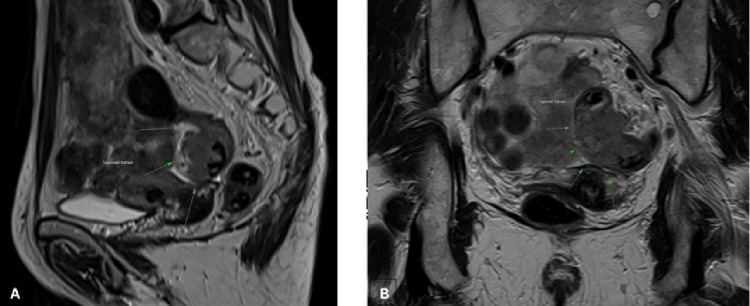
(Case 6) (A) Sagittal view, (B) Coronal view: MRI pelvis showing 4 cm sigmoid colon tumour (Green arrows).
